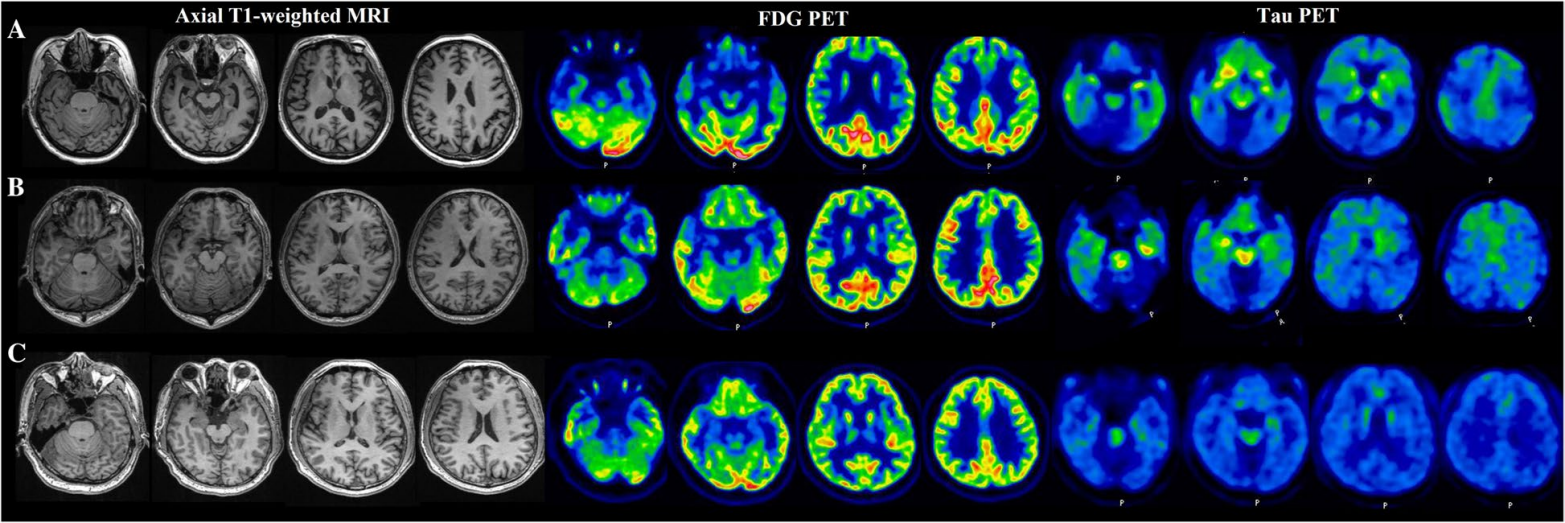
Neuroimaging presentations of the svPPA patient and two presymptomatic carriers. A IV-22 (svPPA patient): bilateral frontal atrophy and bilateral but left-dominant atrophy of the anterior temporal lobes on brain MRI; hypometabolism in the bilateral temporal cortex, frontal cortex, insula and caudate nucleus on FDG PET; and diffuse tracer binding in the temporal and frontal lobes of the cerebrum on tau-PET. B IV-2 (presymptomatic carrier with abnormal cognitive assessment): no visually obvious atrophy of the bilateral frontal and temporal lobes on brain MRI; focal hypometabolism of the bilateral frontal lobes on FDG PET; diffuse tracer binding in the temporal and frontal lobes of the cerebrum on tau-PET. C IV-30 (presymptomatic carrier with normal cognitive assessment): no obvious atrophy, hypometabolism or tau accumulation on brain MRI and PET scans. There is “off-target” binding in brain areas without tau deposition, such as the brain stem and the basal ganglia
